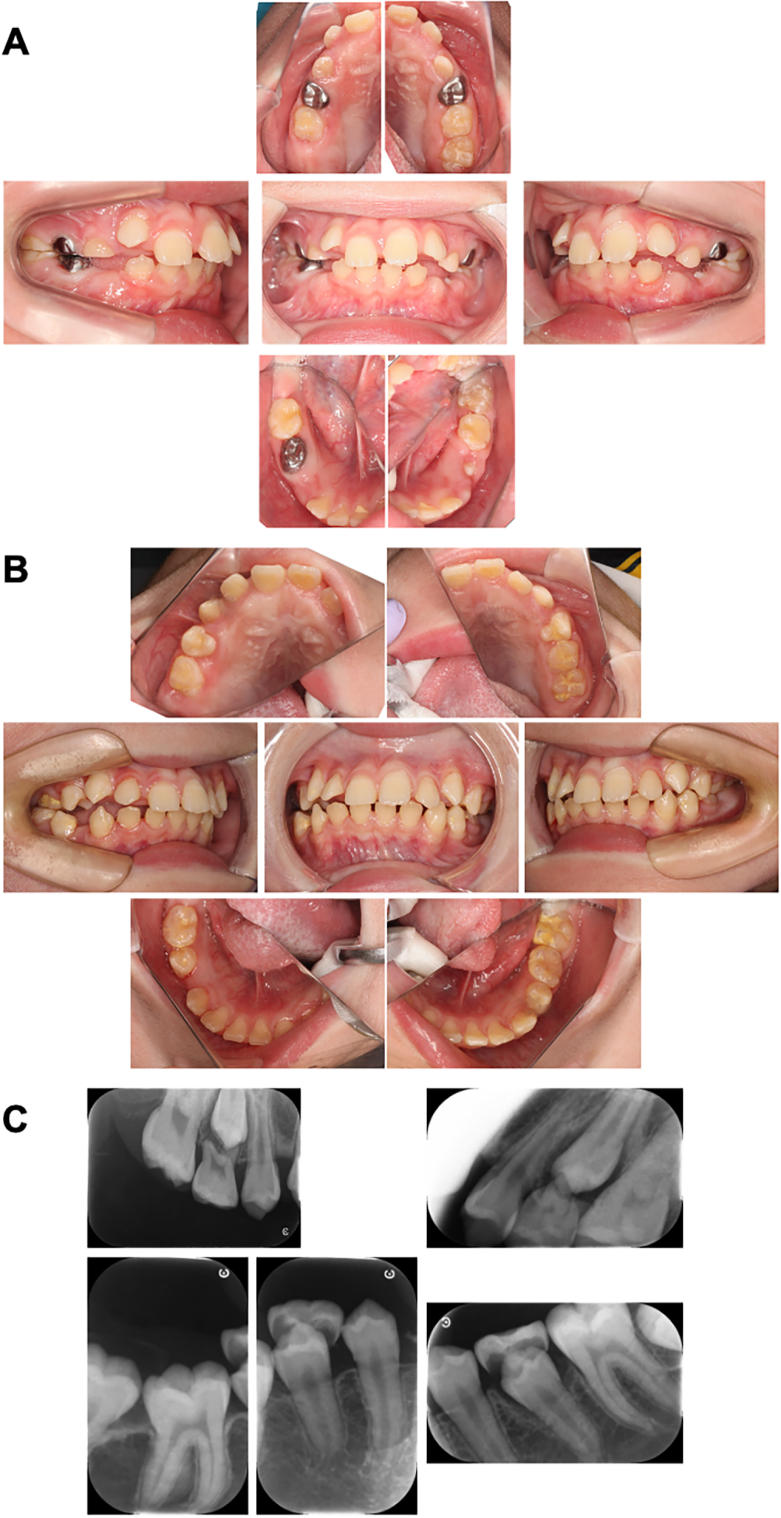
Intraoral photographs at the time of periodic medical check-ups. (A) Intraoral photographs at the time of periodic medical check-up at 9 years and 6 months-old. There are no caries and the oral hygiene was improved in this case. (B) Intraoral photographs at the time of periodic medical check-up at 11 years and 10 months-old. The primary first molars were replaced with permanent teeth. (C) Dental x-ray image at the time of periodic medical check-up at 11 years and 10 months-old.
